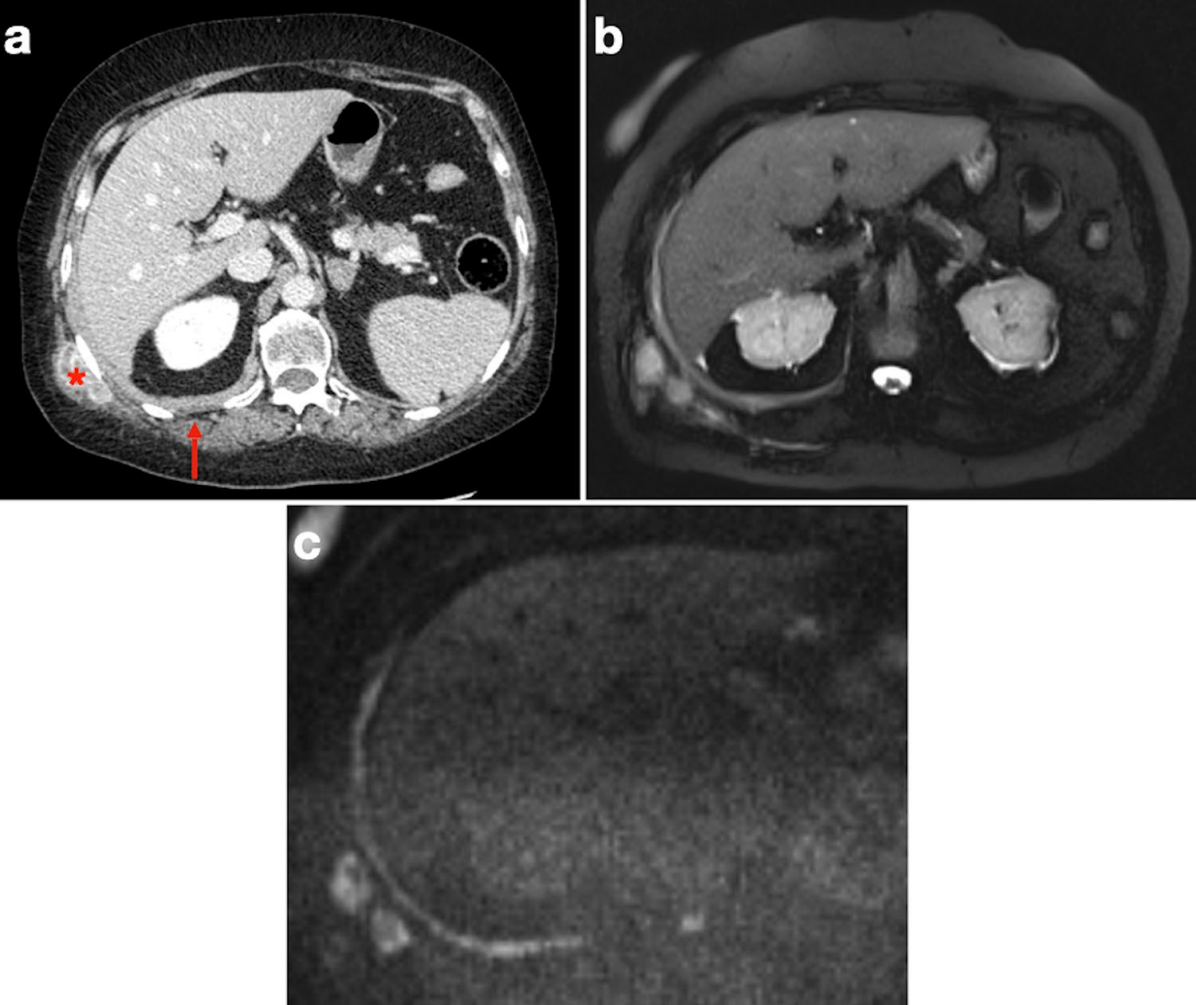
70-year-old man with history of asbestos exposure and known PM under chemotherapy. a Axial CT image shows irregular thickening with contrast enhancement of the posterior costophrenic sulcus (arrow) and nodular mass along the outer aspect of the right tenth rib, as a result of tumor seeding from previous percutaneous biopsy. b Axial fat-suppressed T2-weighted and c diffusion-weighted images clearly depict tumor deposits with sharp tumor-to-normal-tissue contrast
